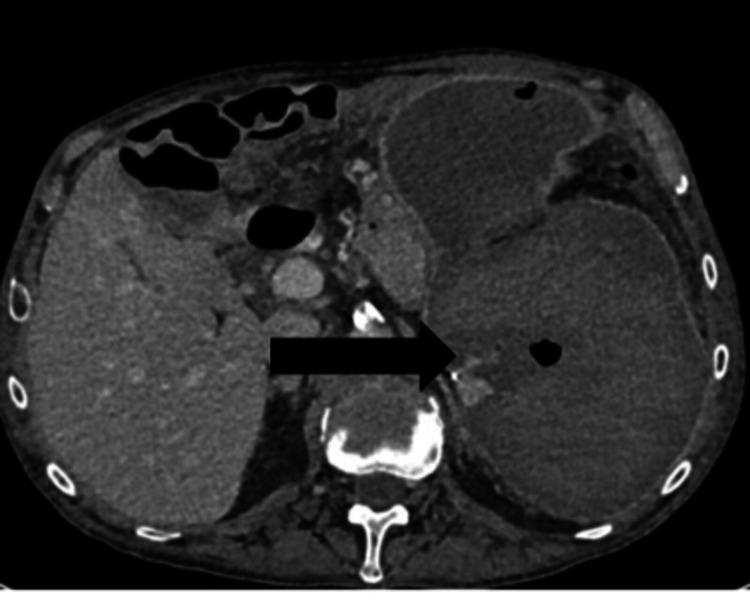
CT abdomen showing gas locules in the large splenic hematoma
